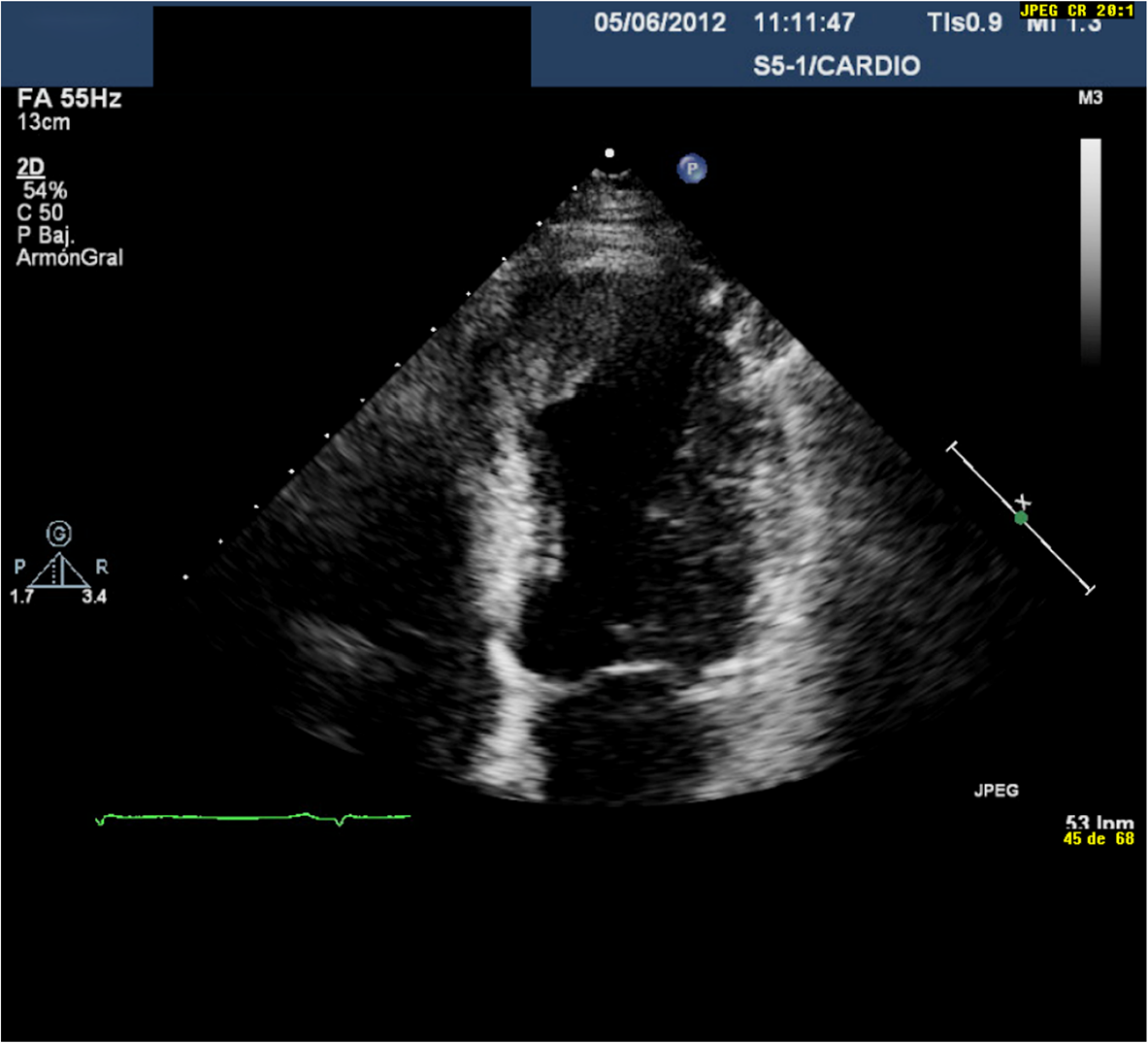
At 6 months after discharge, transthoracic echocardiography showed recurrence of apical thrombus, and the same abnormalities of left ventricular segmental contraction observed at 4 months after discharge.
